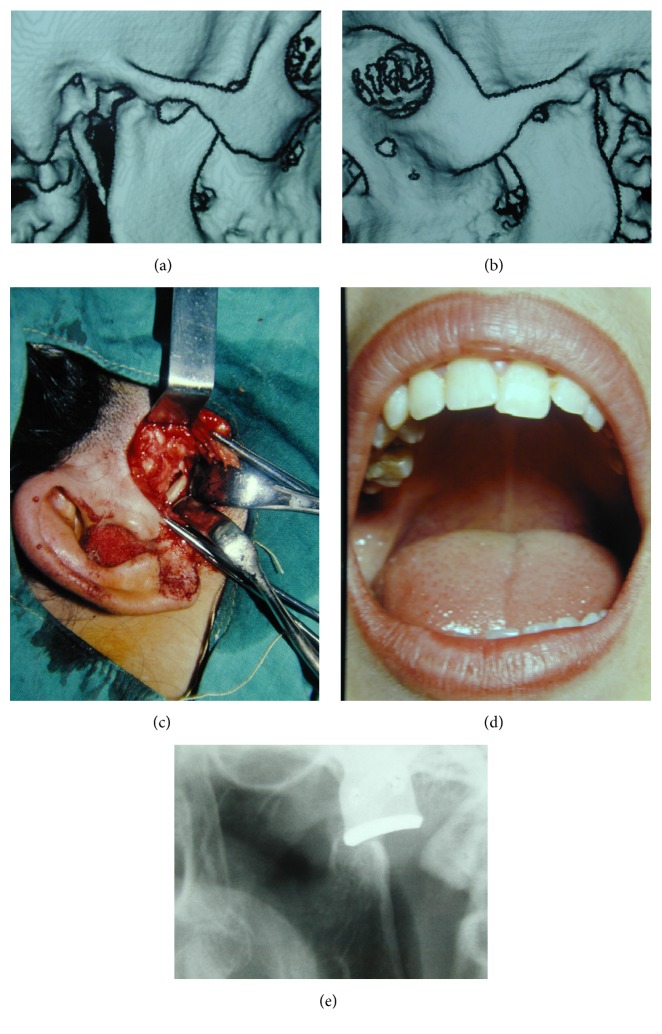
(a) During the examination of the patient (Case  2), CT revealed a spontaneously healed condyle after having a fracture at the right side. (b) Ankylosis at the left side (Case  2). (c) Horizontal resection of the ramus (Case  2). (d) Mouth opening provided by surgical treatment (Case  2). (e) Panorex shows a spontaneously healed condylar fracture at the right side and new condyle formation after surgical treatment of ankylosis at the left side (Case  2).
